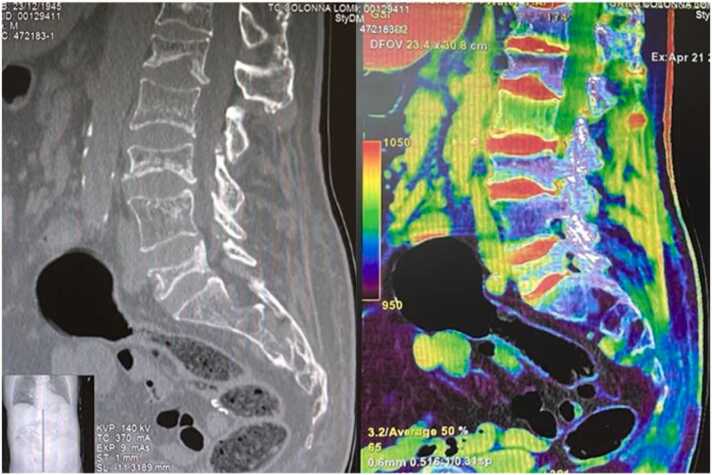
Comparison of the same patient complaining of low back pain and a history of multiple VCFs. On the left, the sagittal CT scan shows multiple VCFs, leaving doubts about which are the acute VCFs. On the right image acquired by the dual energy technique, vertebral bodies are colored in green when bone edema is present, as in acute fractures, and in blue in healed fractures. The picture outlines acute fractures of L2 and L5, and healed fractures of T12, L1, L3, and L4.
